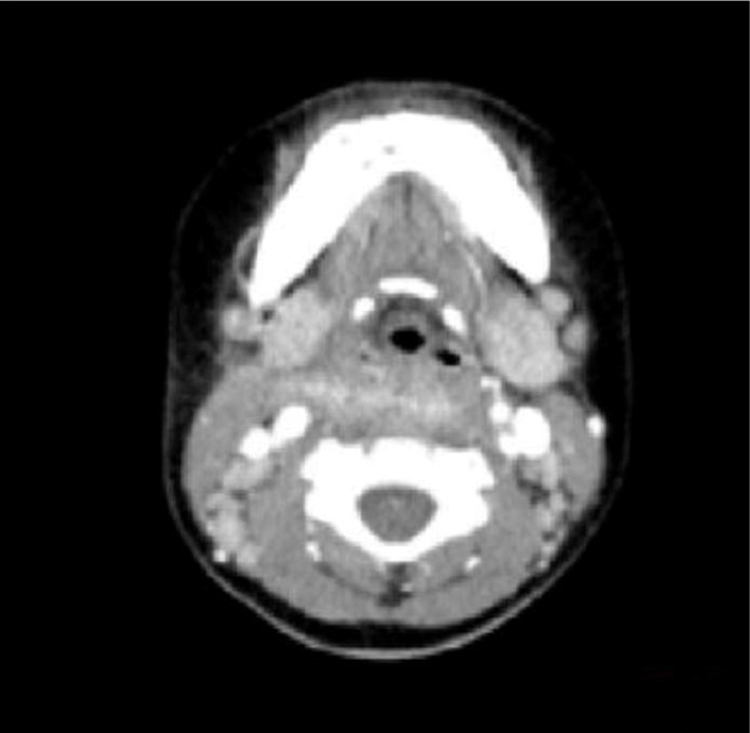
Review of the neck CT scan, the neck soft tissue is slightly swollen.
